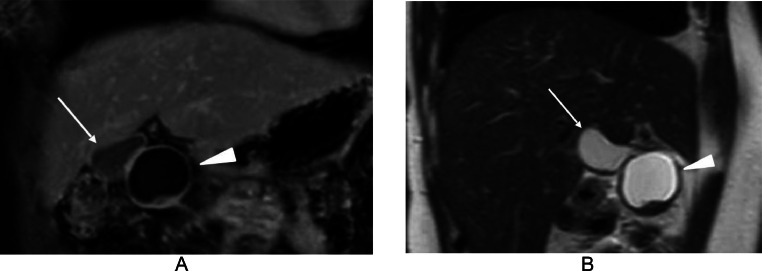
Magnetic resonance imaging (MRI) findings. (A) Coronal view MRI of both the gallbladder (arrow) and intracystic tumor (arrowhead) contents showed a hypo-intense pattern on T1-weighted images. The latter intensity was much lower than that of the former. (B) Sagittal view MRI of the 2 contents showed a hyper-intense pattern on T2-weighted images. The latter intensity was somewhat higher than that of the former.
